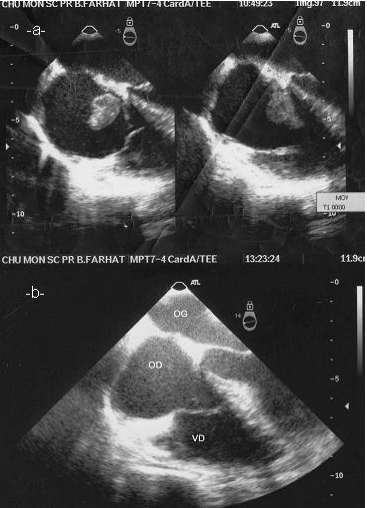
Transthoracic echocardiography: image of the thrombus in the right atrium (a), after treatment complete resolution of the thrombus (b).
